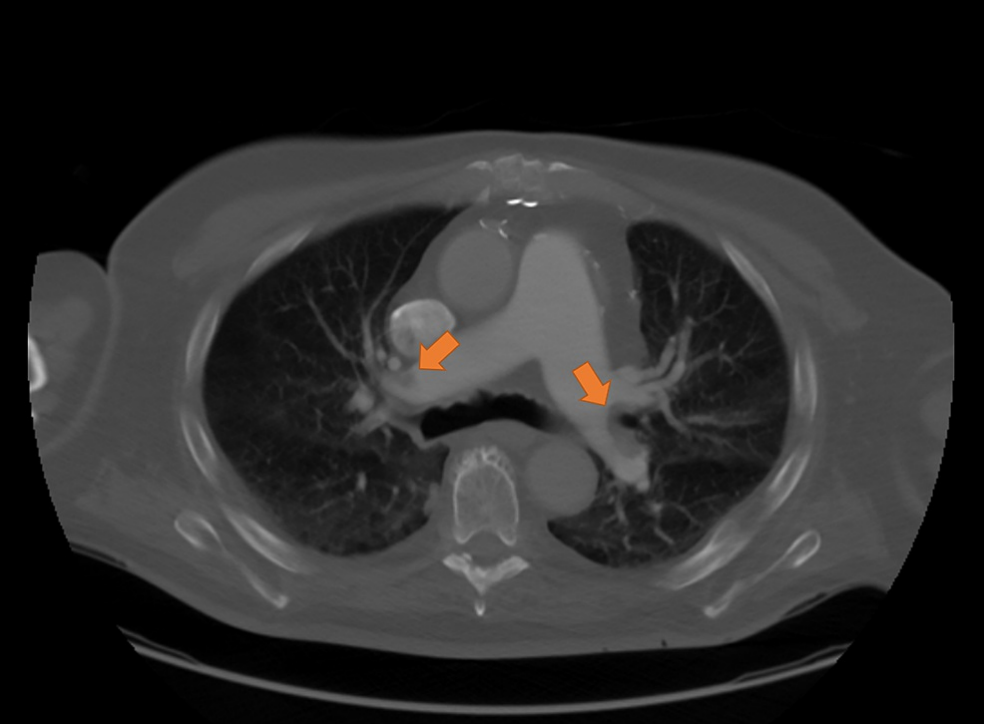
CT chest following tPA therapy showing reduced clot burden compared to the previous exam (arrows) CT: computed tomography; tPA: tissue plasminogen activator
